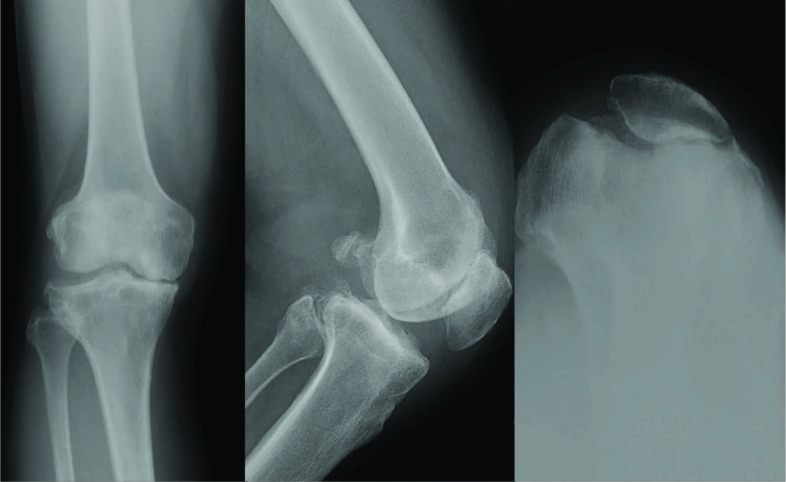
The radiographs present a patient, who is not a suitable candidate for UKA due to bone on bone retropatellar osteoarthritis despite medial osteoarthritis and intact ACL.
